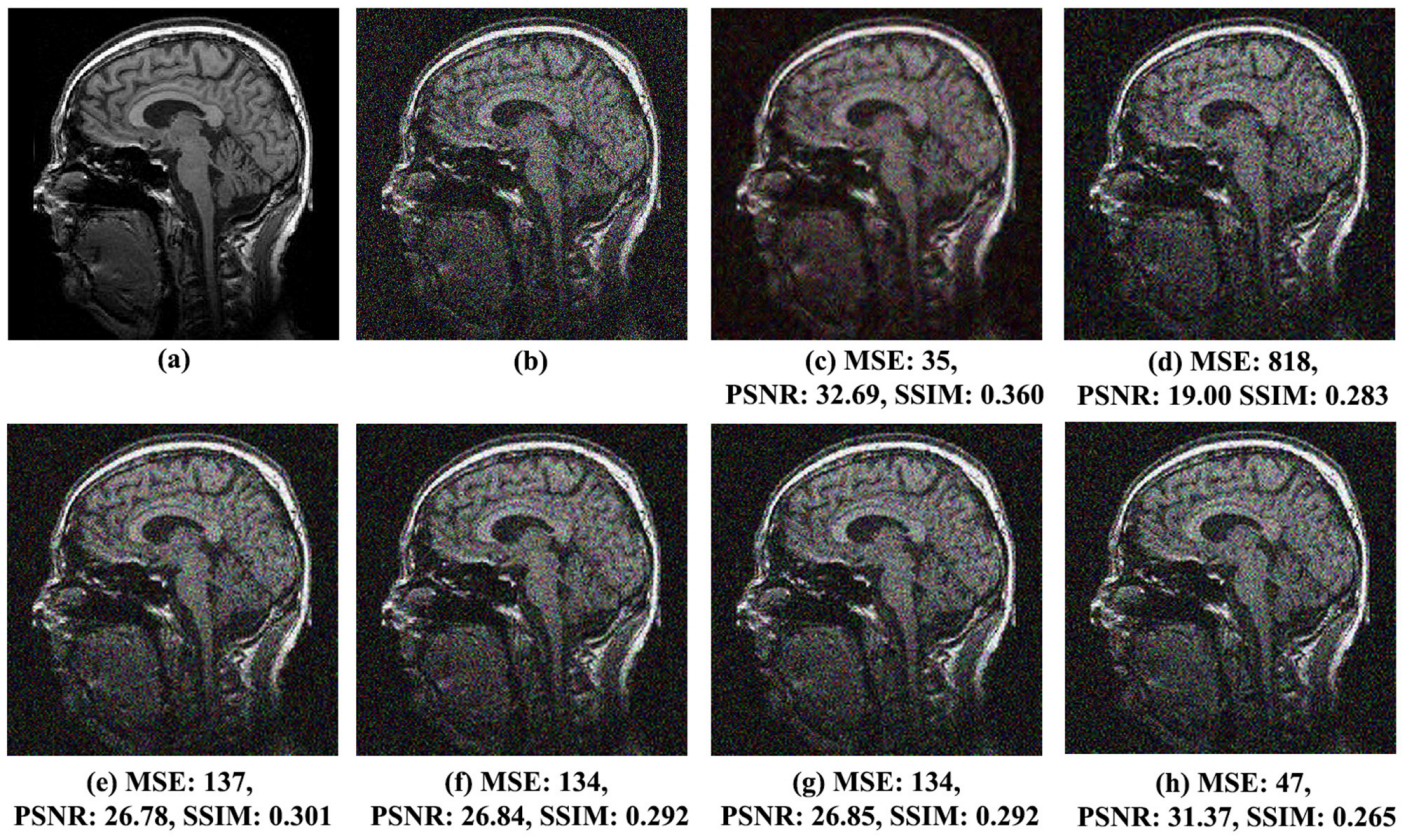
Comparison of noise reduction performance for MRI Brain Image 6 with noise variance of 0.05: (A) original image, (B) noisy image, (C) proposed method, (D) VisuShrink, (E) BayesShrink, (F) Gaussian Kernel, (G) improved threshold, and (H) AGGD.
